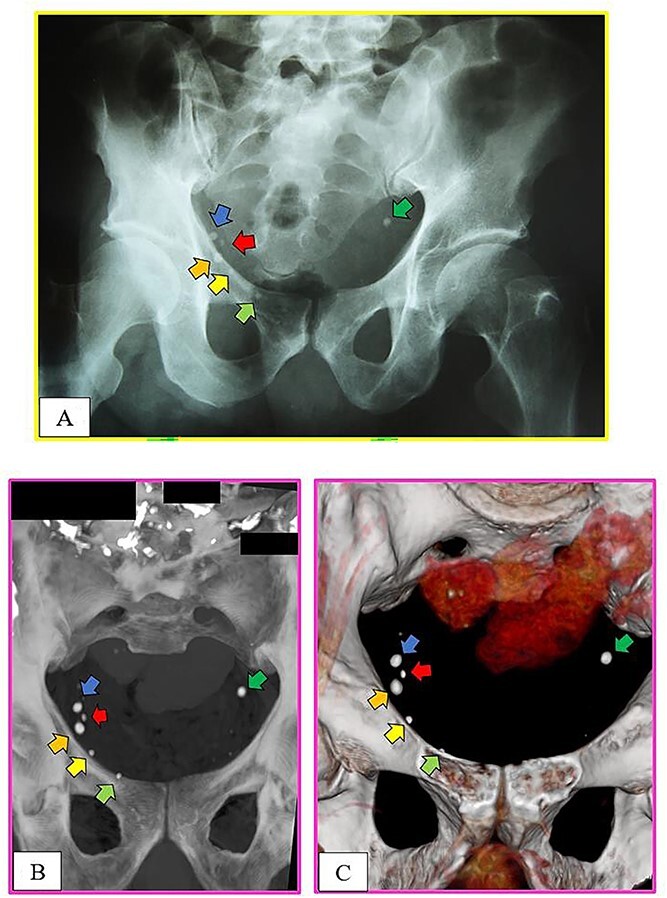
Comparisons of the X-ray and computed tomography (CT) images. Pelvic calcifications. Intravitam X-ray (A), conventional postmortem CT (B), and postmortem CT with 3D reconstruction (C).
